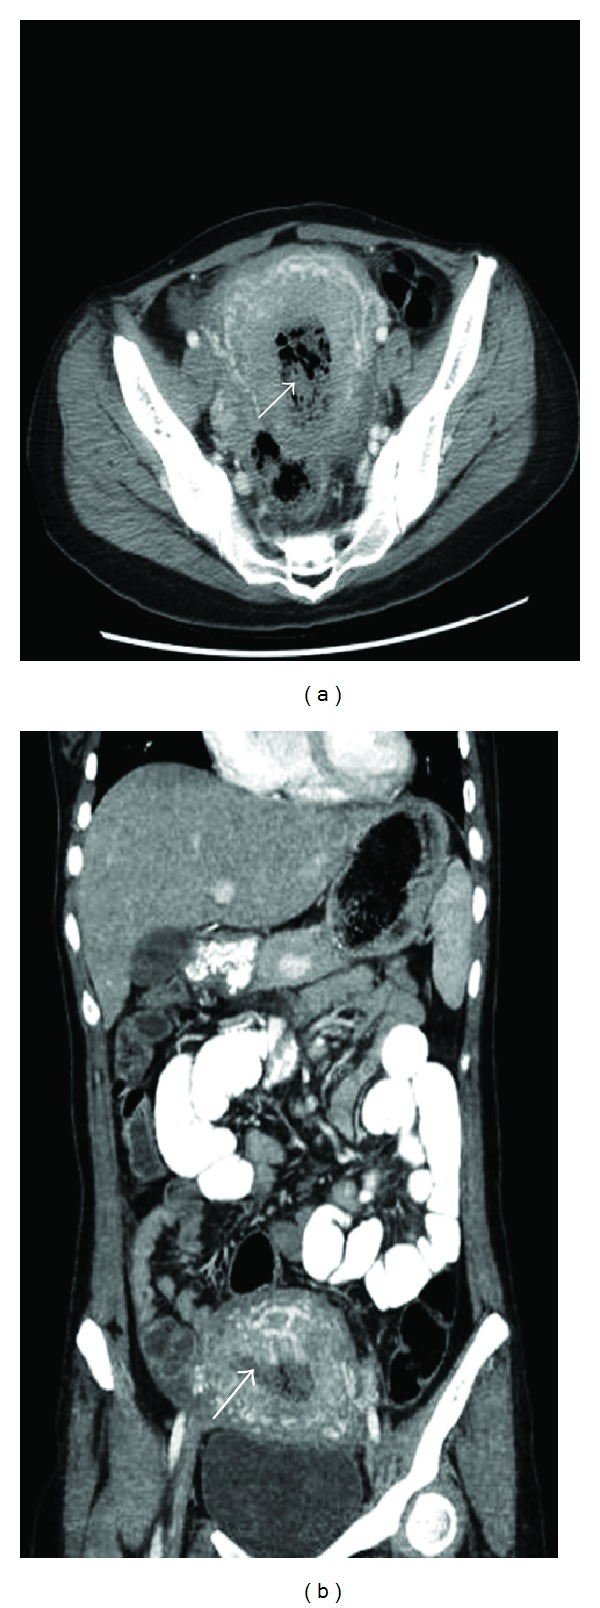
CT scan of abdomen and pelvis. Enlarged uterus with a markedly distended endometrial cavity contained a heterogeneous collection of soft tissue, debris, and gas bubbles (arrow).
